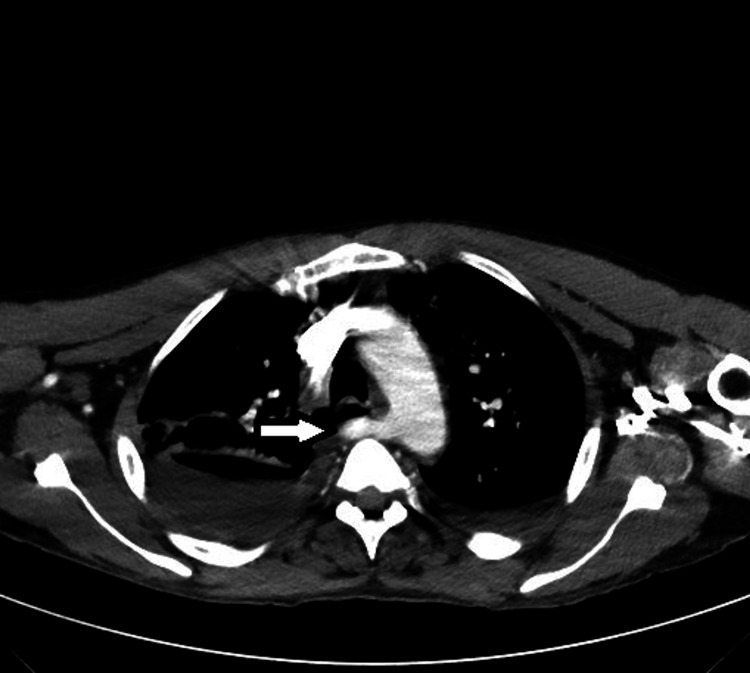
Chest CT angiography Chest CT angiography showing an aberrant right subclavian artery (arrow) arising from the right subclavian artery
